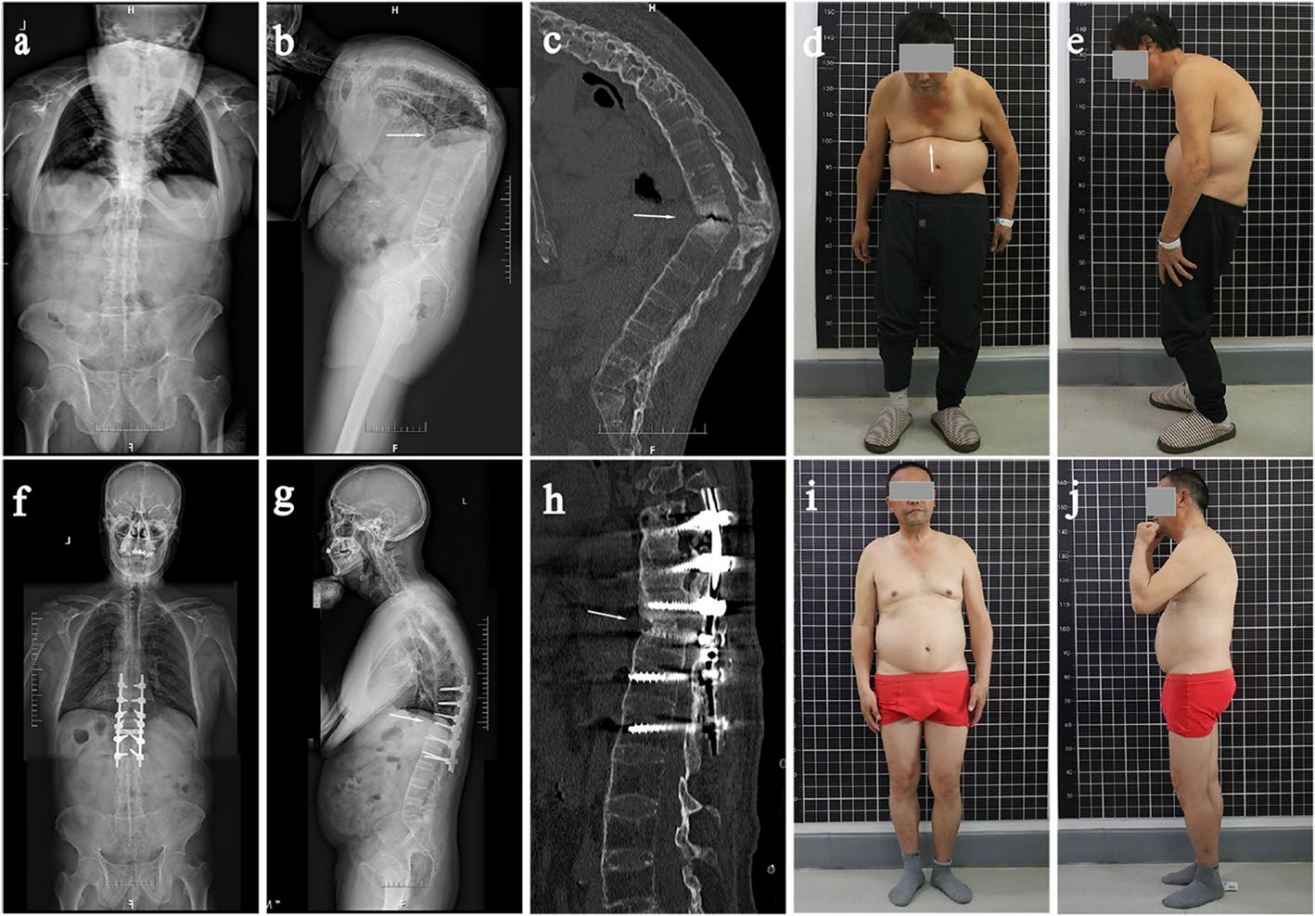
A 58-year-old male patient, diagnosed with AS combined with T11/12 AL. a Preoperative full length anteroposterior radiograph of spine. b Preoperative lateral spinal radiographs showed excessive thoracic kyphosis and a T11/12 intervertebral fracture line (white arrow). c Preoperative CT sagittal view showed T11/12 intervertebral space involving a three-column fracture and a visible vacuum sign (white arrow). d, e Preoperative gross image showing severe kyphosis of the spine with abdominal wall wrinkles (white arrow); f Full length anteroposterior radiographs of the spine 12 months after surgery. g Full length lateral spinal radiographs 12 months after surgery showed normal thoracic kyphosis. h Sagittal CT images at 12 months postoperatively showed intervertebral fusion with the bone graft. i, j Postoperative gross radiography showed normal physiological curvature of the thoracolumbar spine, and the appearance was significantly improved
